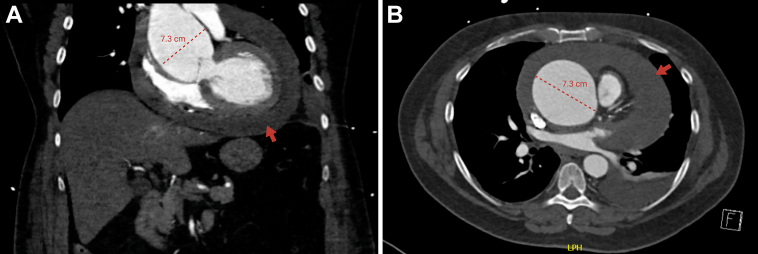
Preoperative Contrast-Enhanced Computed Tomography (A) Coronal and (B) axial views demonstrating a large ascending aortic aneurysm (7.3 cm) with an associated pericardial effusion (red arrow).
